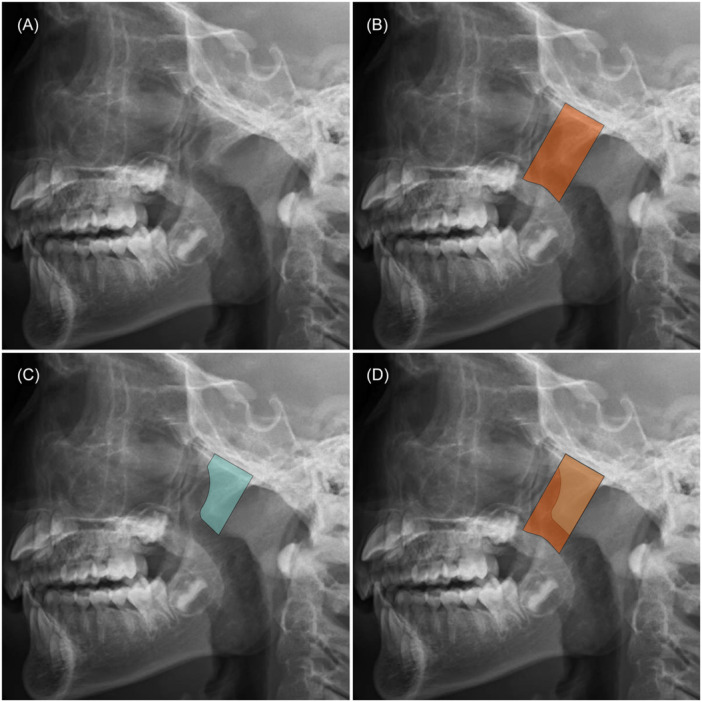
Methodology for calculating the ANAR in lateral cephalometric radiography. (A) original lateral nasopharyngeal radiographs. (B) Anterior area of nasopharynx. (C) Anterior area of adenoid. (D) ANAR. ANAR, adenoid/nasopharynx area ratio.
